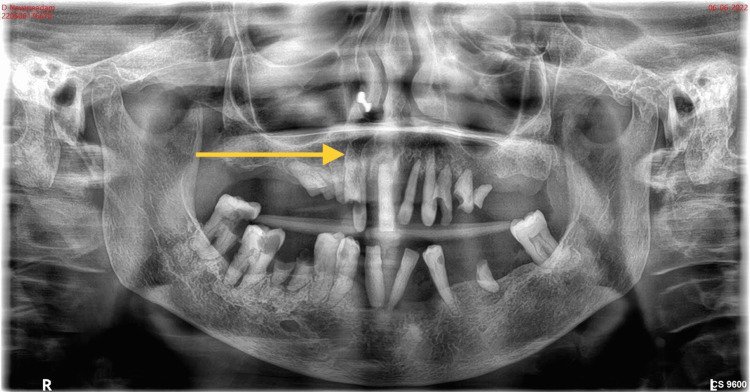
Pre-operative radiograph Represents the orthopantomogram showing the generalized horizontal bone loss in the upper arch and root stumps 13, 14, 15, 23, 24, 25, 36, and 46. Dental caries involving pulp in 47, 48, and 44 approximating pulp in 12 and 34. The arrow mark shows the generalized horizontal bone loss in the maxilla.
